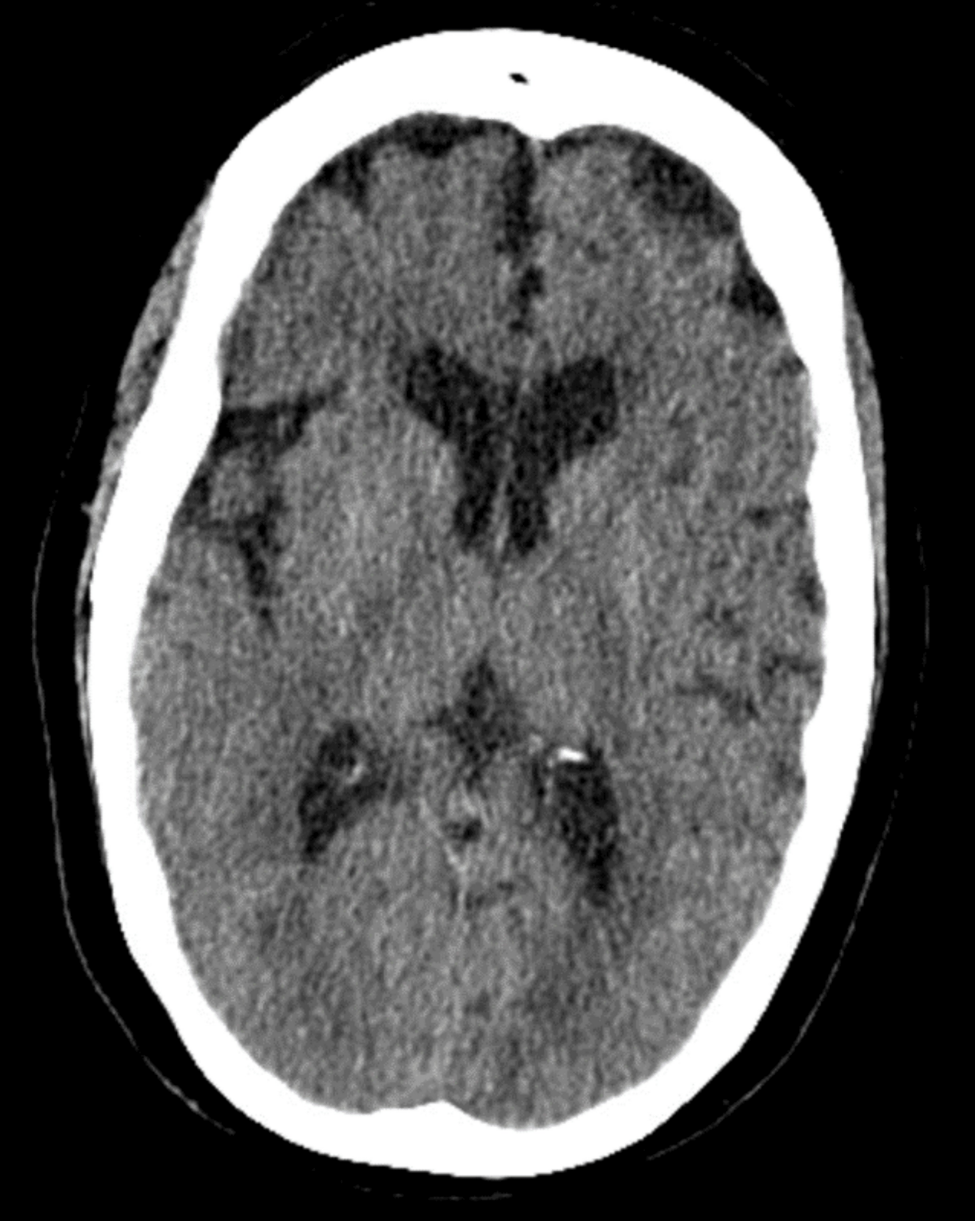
Representative axial section of a normal CT scan of the head CT: computed tomography
